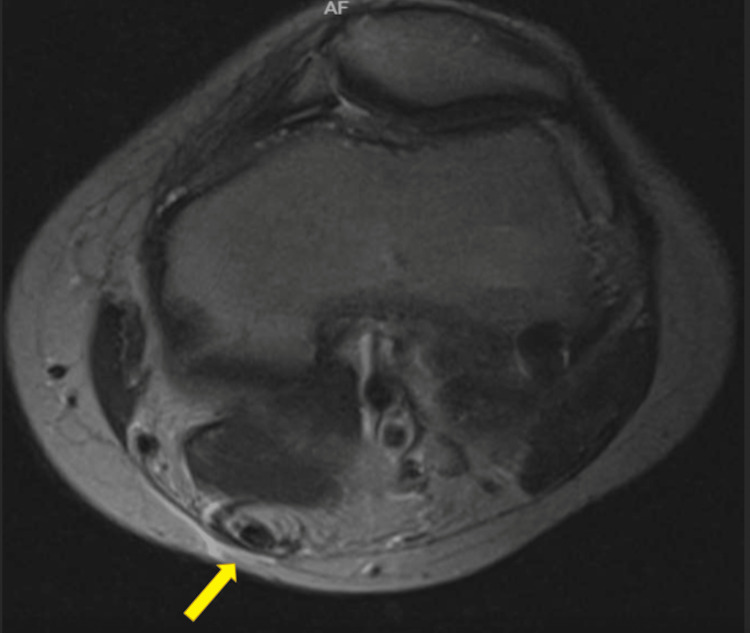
Axial T2 magnetic resonance imaging: Distal third left thigh level. The left semitendinosus tendon (yellow arrow) is slightly thickened and inhomogeneous. A small fluid collection surrounds the tendon.
